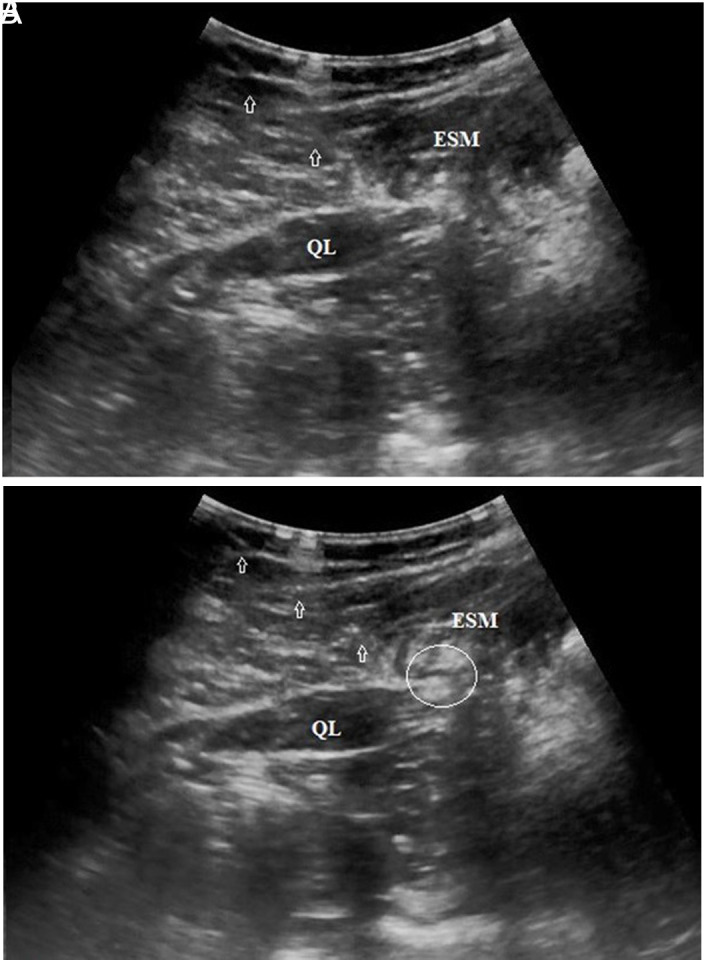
Ultrasound imaging interfascial injection. 2A, 2B: QL: quadratus lumborum muscle, ESM: erector spinae muscle, white arrows show block needle. 2B: white circle injection site.
